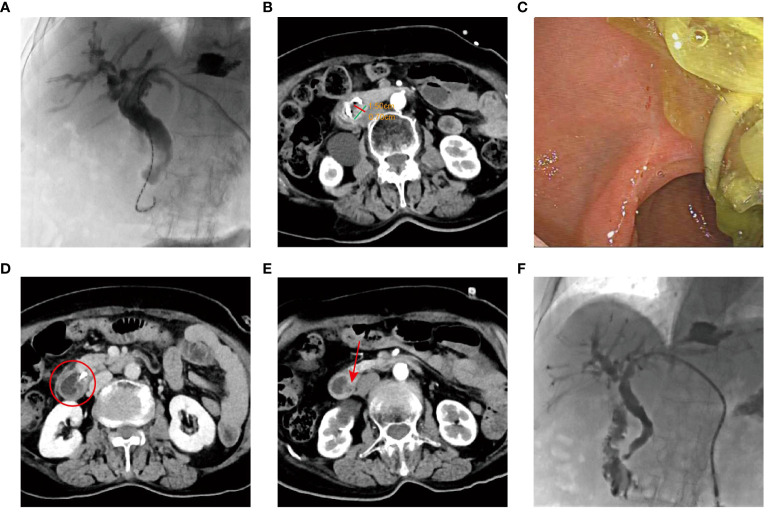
The examination results of Case 1 after ^125^I implantation. (A) Placement of ^125^I seed strands on the tumor surface. (B) Three months after ^125^I seed implantation, enhanced CT indicated the treatment response was PR. (C) Three months after brachytherapy, duodenoscope revealing disappearance of the lesions, with no duodenal obstruction. (D) Eight months after ^125^I seed implantation, tumor disappeared (the red circle). (E) Ten months after ^125^I seed implantation, no recurrence (the arrow indicates the duodenal papilla). (F) The images of duodenography and cholangiography.
